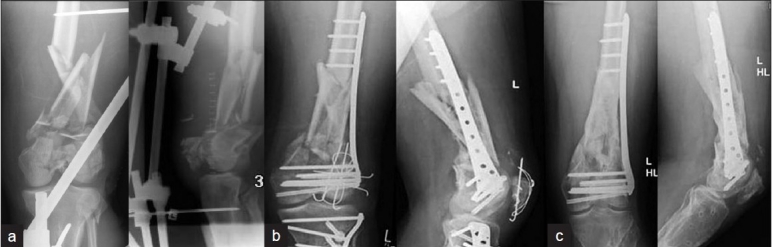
(a) Anteroposterior and lateral radiographs of left knee of a 38-year-old man with type C3 open distal femur fracture, patella fracture and proximal tibial fracture on the left leg; initial treatment with external fixator and temporary vacuum assisted closure. (b) Anteroposterior and lateral X-rays show definitive treatment with LISS plate after conditioning of soft tissues. (c) Nonunion developed and was treated with re-osteosynthesis, and application of osteogenic protein 1 (OP1, bone morphogenetic protein-7 (rhBMP-7)), and cancellous bone that resulted in osseous union. The Patellar and upper tibial implants were removed
